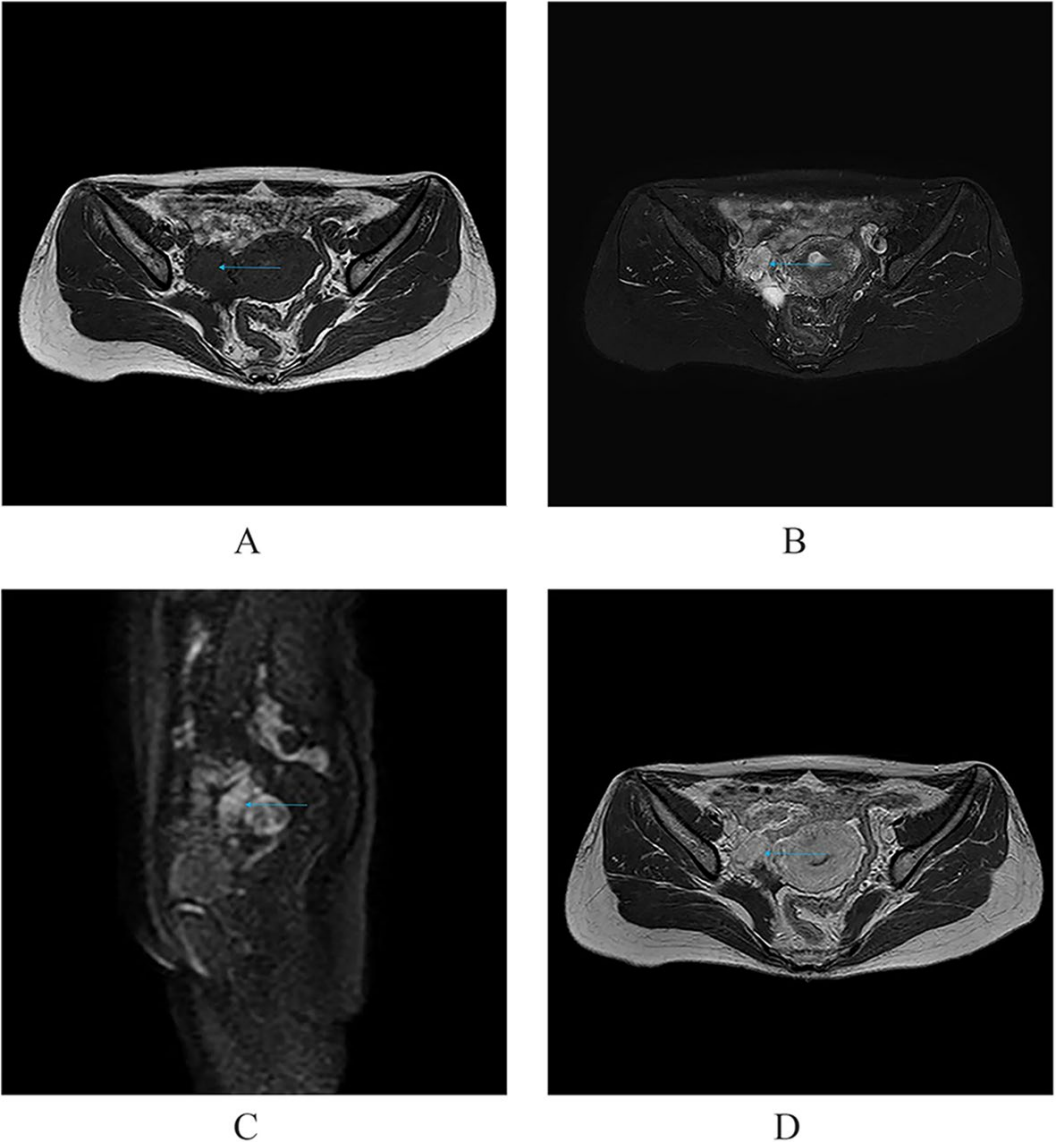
Limited OMM: Isointense on T1WI and muscular tissue, and markedly hyperintense on T2WI and DWI, which is significantly enhanced after enhancement. A linear “capsule” (long arrow) can be seen around it
